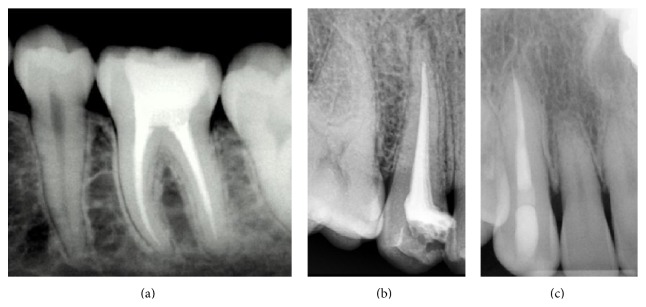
Radiographs depicting adequate quality root canal (a), inadequate/short length (b), and inadequate/overextended length (c).
